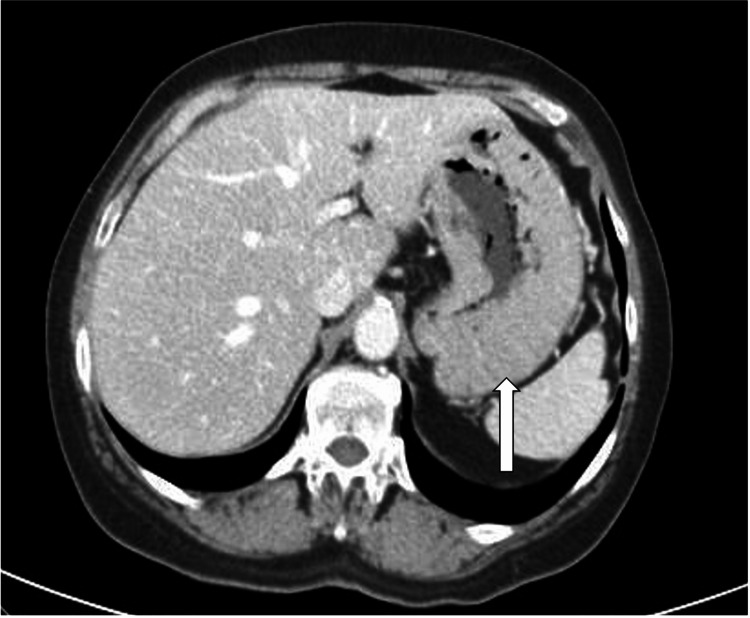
Abdominal CT scan showing gastric wall thickening
